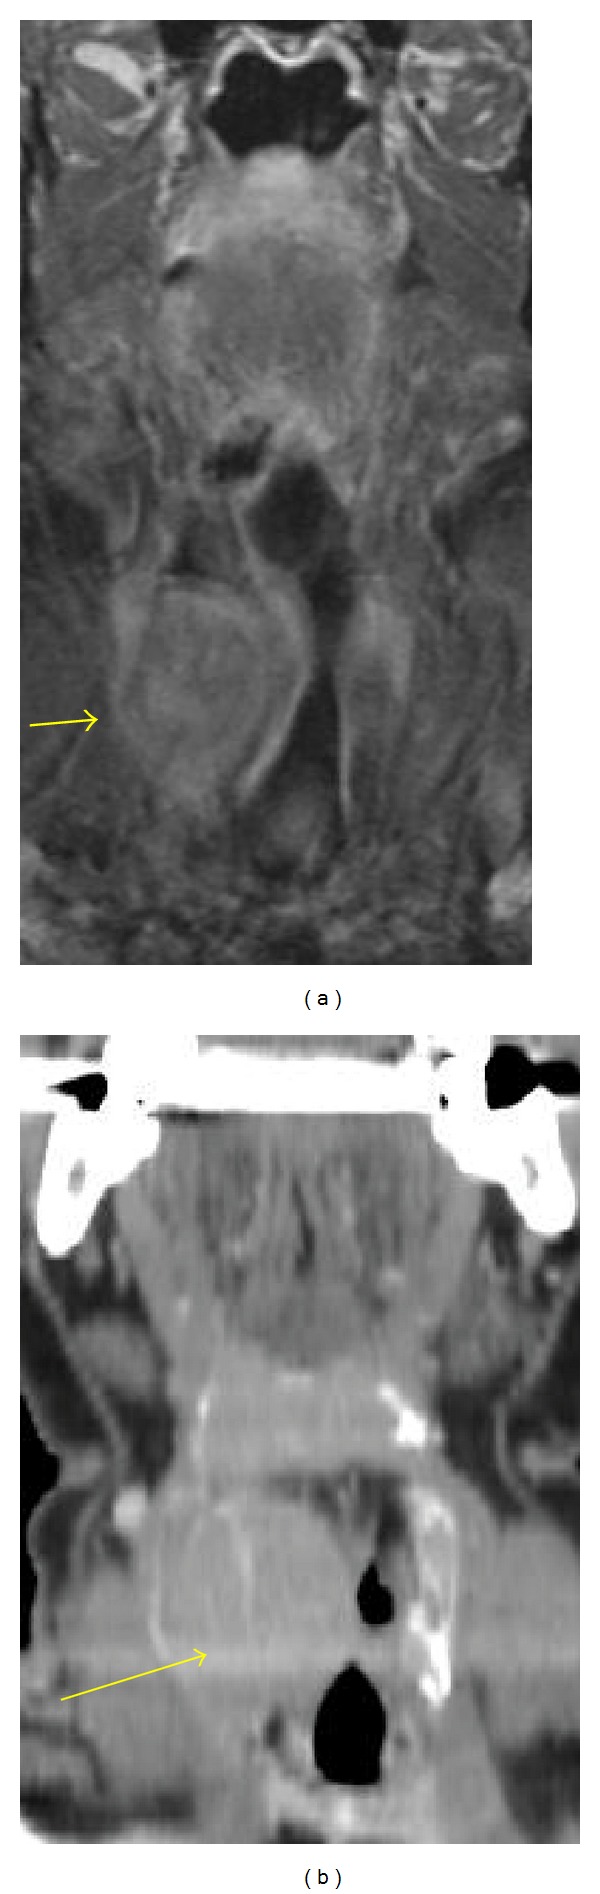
Coronal MRI (a) and coronal CT (b); postcontrast homogeneous enhancement of the mass.
